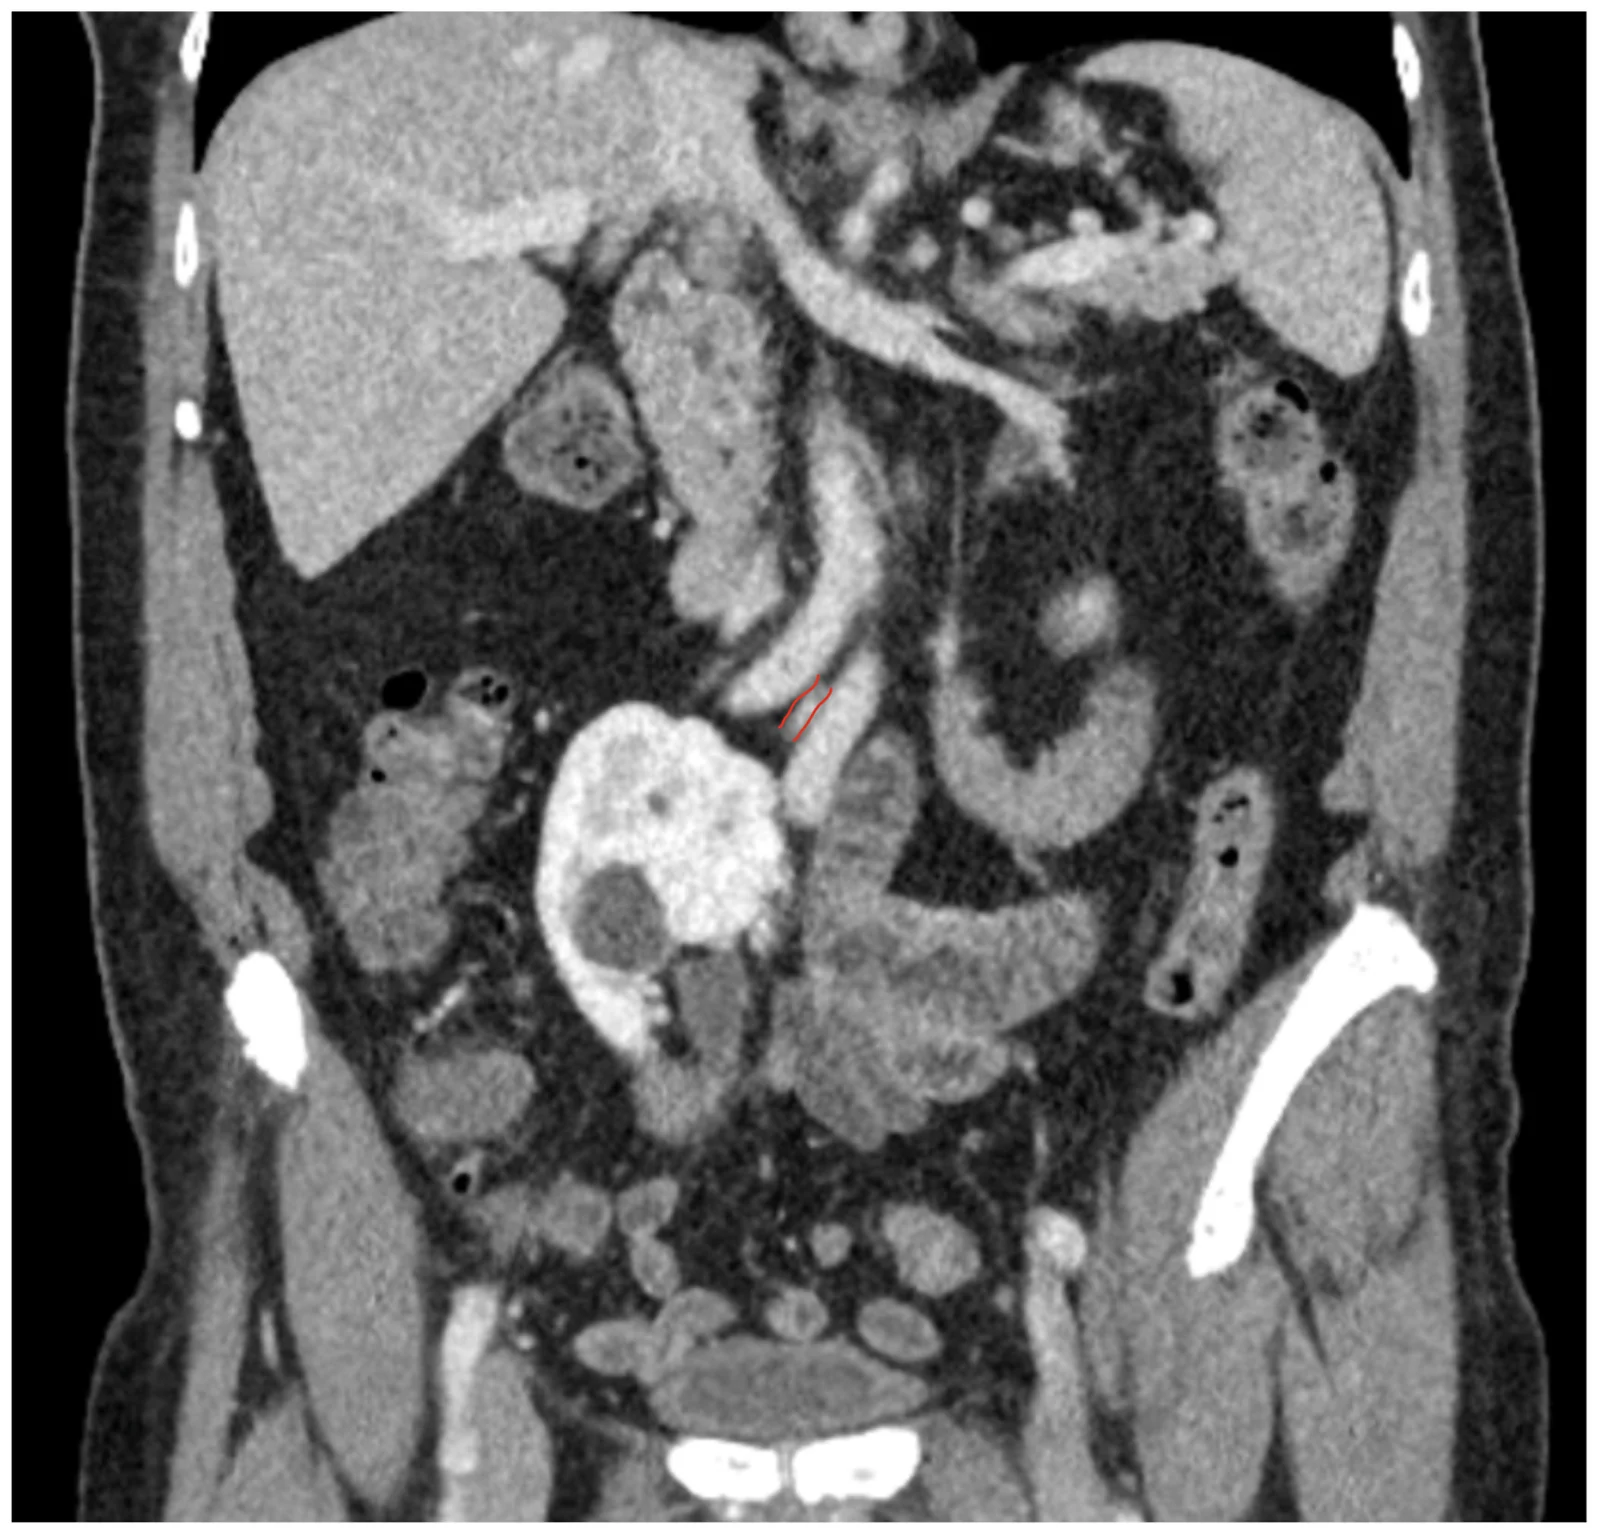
Contrast-enhanced computed tomography (CT) urogram slices with the right renal artery highlighted in *red*, demonstrating the relationship of the low-lying malrotated right pelvic kidney to the adjacent right iliac artery.
